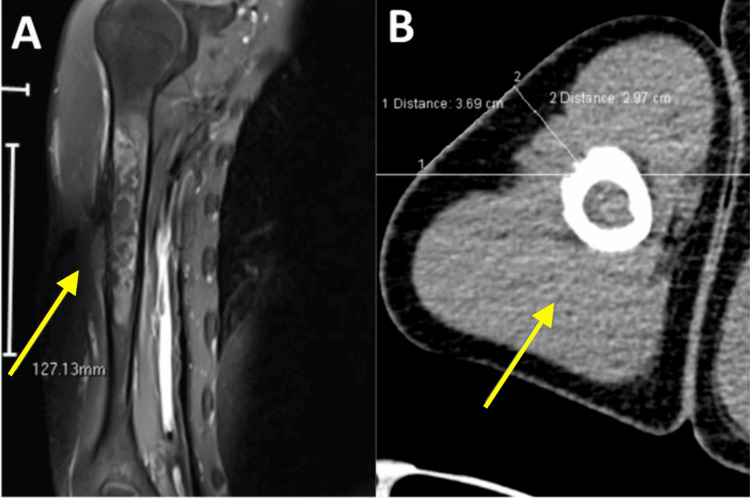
Magnetic resonance imaging (MRI) scan revealed a lytic necrotic lesion characterized by hyperintense signal intensity on T2-weighted images in the right humerus (arrow). Sagittal (A) and axial views ( B).
